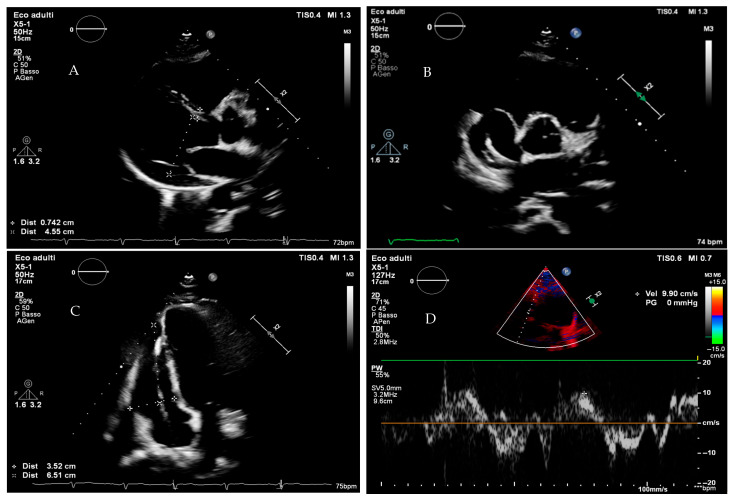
Transthoracic echocardiographic images from a woman who presented with sustained ventricular tachycardia during pregnancy. Subsequent evaluation revealed a pathogenic variant in a desmosomal gene, leading to the diagnosis of arrhythmogenic cardiomyopathy. Left ventricular systolic function is preserved. (Panel A): Parasternal long-axis view showing normal left ventricular wall thickness and chamber dimensions. (Panel B): Parasternal short-axis view showing right ventricular dilation. (Panel C): The right ventricle appears mildly dilated (The line between the asterisks), with reduced tissue Doppler velocities (Panel D).
